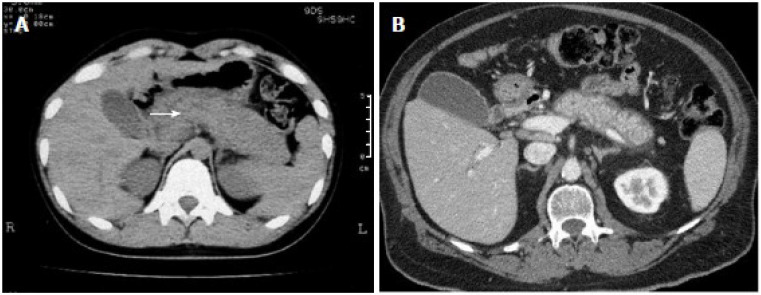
Characteristic Imaging Findings of AIP-1. (A) Diffuse pancreatic enlargement with the characteristic sausage-shaped appearance can be seen (arrow). (B) The peripancreatic rim seen around the body and tail of the pancreas is a key radiographic finding of AIP [52].
